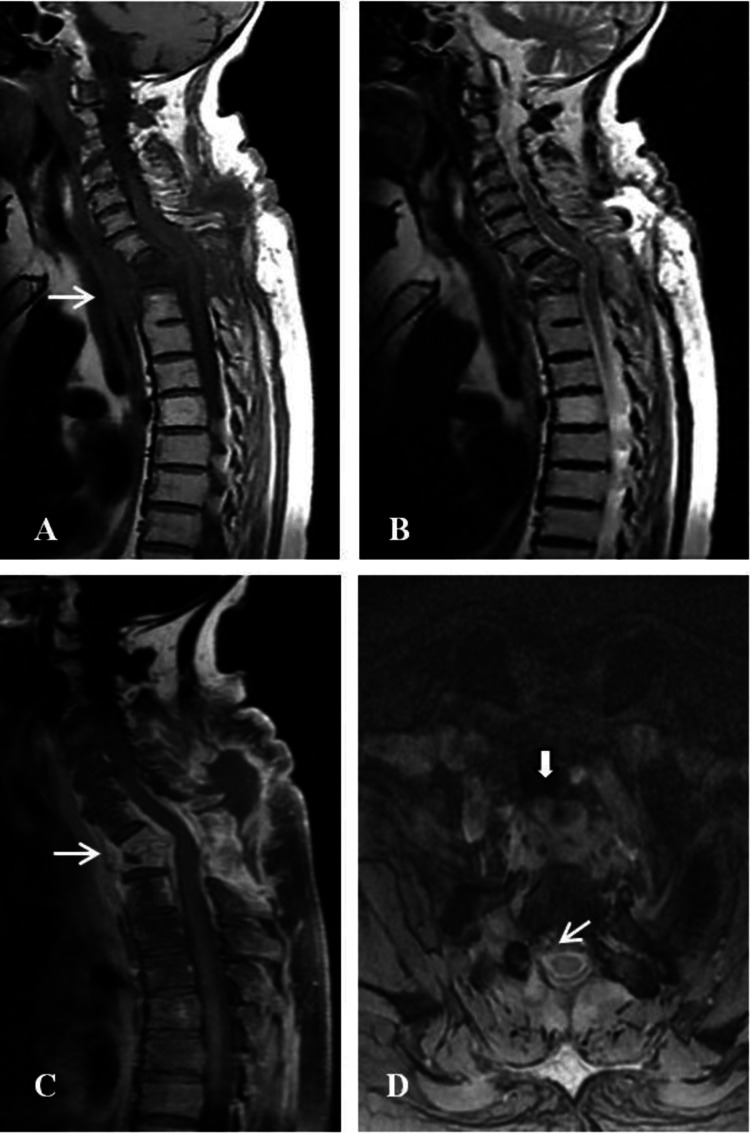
MRI of the patient with tuberculous spondylodiscitis at the T1 – T2 level (Case 1) (A) Sagittal T1 projection – hypointense signal with subligamentous spread and presence of paravertebral abscess (thin solid arrow); (B) Sagittal T2 projection – inhomogeneous hyperintense signal; (C) Sagittal T1 projection with contrast – focal inhomogeneity and enhancement of certain intraosseous structures and paravertebral abscess (thin solid arrow); (D) Axial T1 projection with contrast – well-defined ventral paravertebral abscess (thick solid arrow) and epidural abscess (thin solid arrow).
